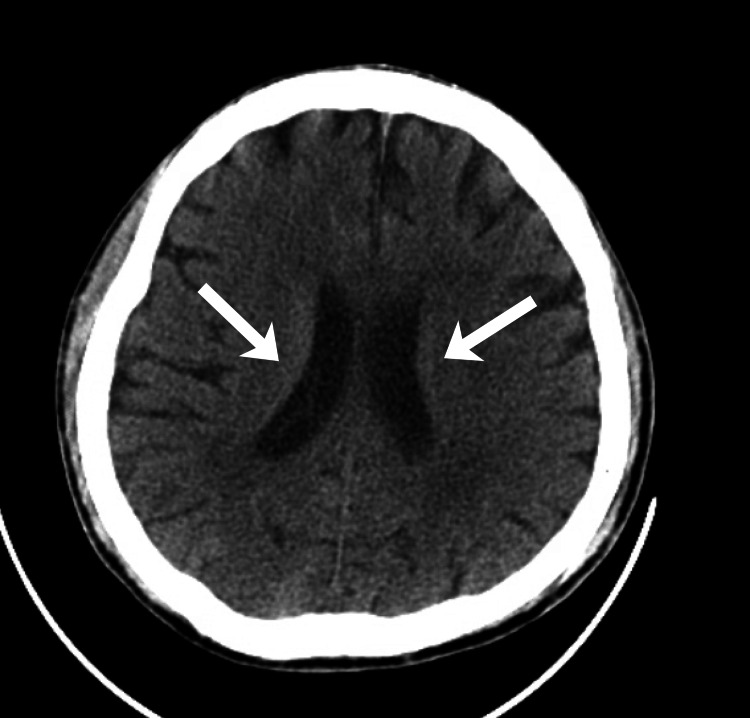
Non-contrast axial CT scan of the head demonstrating normal ventricular size and morphology. The arrows highlight the right and left lateral ventricles, which appear symmetric and without evidence of hemorrhage, mass effect, or cerebral edema. These findings supported the absence of an acute intracranial process contributing to the patient’s altered mental status.
